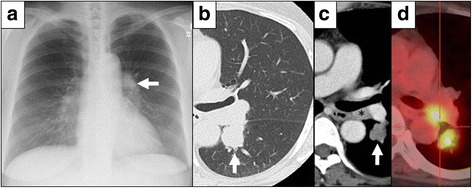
Medical imaging findings and gross appearance of the pulmonary nodule. a An abnormal left hilar shadow was observed (*white arrow*). b, c Contrast-enhanced computed tomography of the chest revealed a solid 28-mm pulmonary nodule at S6 of the left lower lobe (*white arrow*). c Hilar lymphadenopathy adjacent to the pulmonary artery was also found (*asterisk*). d FDG uptake was demonstrated in both the S6 nodule and the hilar lymph node
